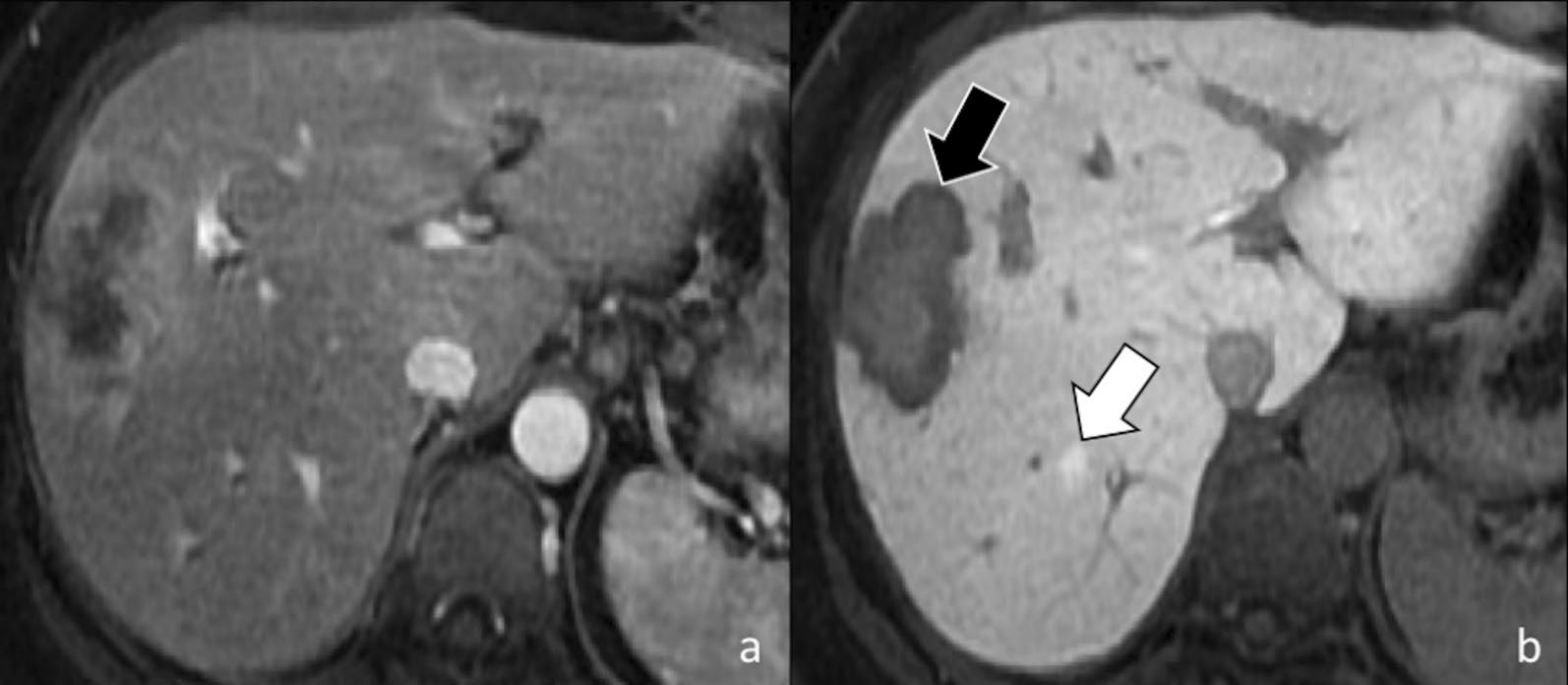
44-year-old woman with breast cancer and liver metastasis treated with chemotherapy. Gadoxetate disodium-enhanced MRI shows a 7 mm regenerative nodule (white arrow) that is not visible in the arterial phase (a), and shows contrast retention in the hepatobiliary phase (b). In the same patient a liver metastasis (black arrow) shows peripheral rim enhancement in the hepatic arterial phase (a) and a target appearance in the hepatobiliary phase (b) with central hyperintensity due to contrast retention and a peripheral hypointense rim
